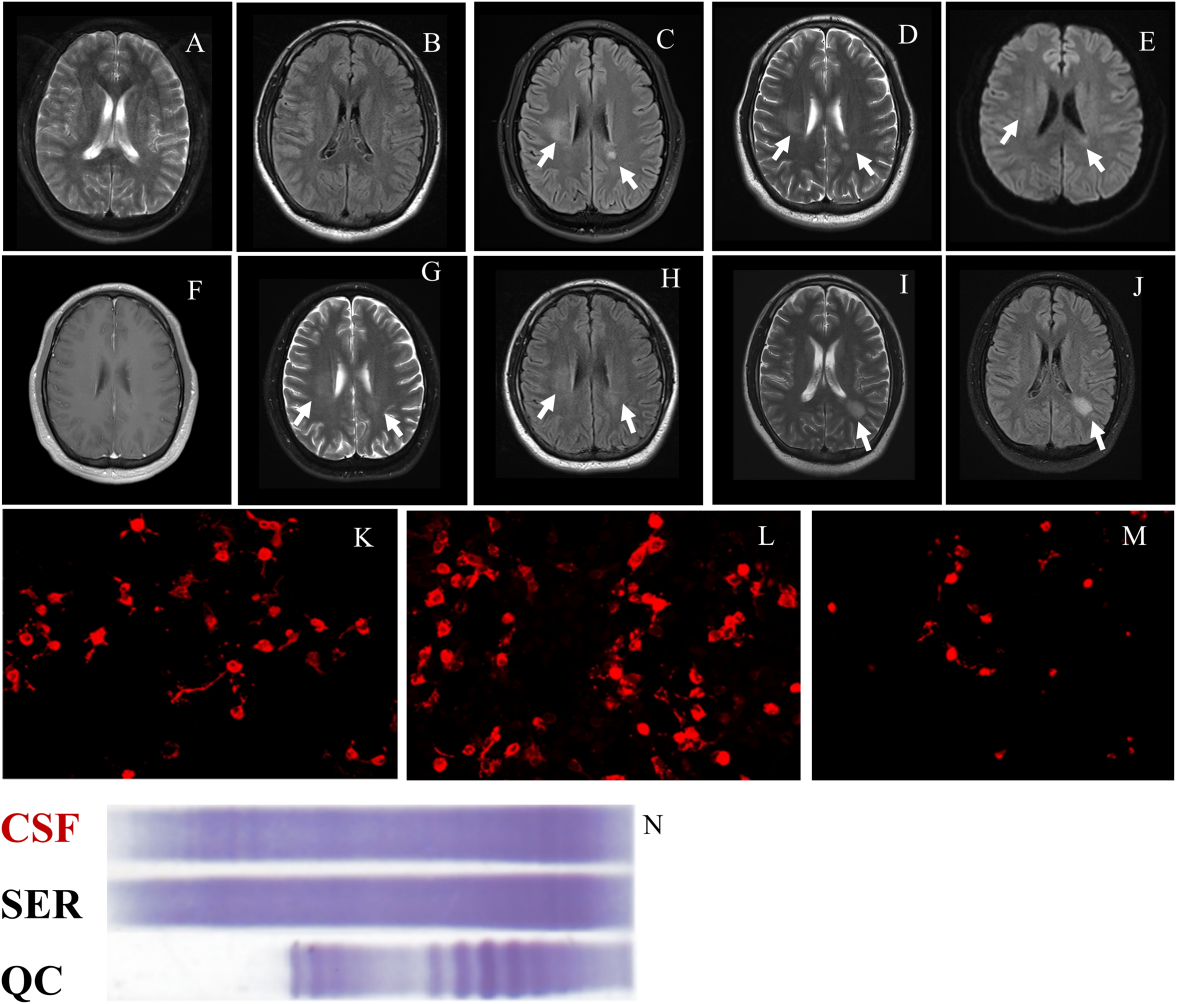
Brain MRI and antibodies in this case. (A) and (B) The MRI performed in October 2021 demonstrated normal results on T2 weight imaging and FLAIR imaging. (C–E) The MRI result showed significant hyperintensity in the right semioval center and the left posterior horn of the lateral ventricle on FLAIR imaging, T2 weight imaging, and DWI on June 1, 2022. (F) Enhanced magnetic resonance imaging performed on June 1, 2022, not showing a significant lesion. (G) and (H) The results of the MRI performed on June 1, 2022 demonstrated that the hyperintensity lesions were alleviated than before on T2 weight imaging and FLAIR imaging. (I) and (J) The results of the MRI performed on October 1, 2022 showed a new lesion with hyperintensity in the left posterior horn of the lateral ventricle on T2 weight imaging and FLAIR imaging. (K, L) Anti-NMDAR antibodies were found to be positive in serum (1:100) and in CSF (1:30) on June 1, 2022. (M) Anti-NMDAR antibodies were found to be positive in CSF (1:10). (N) OB was positive in CSF (counts, ≥2). CSF, cerebrospinal fluid; DWI, diffusion-weighted imaging; FLAIR, fluid-attenuated inversion recovery; MRI, magnetic resonance imaging; OB, oligoclonal immunoglobulin bands; anti-NMDAR, anti-N-methyl-D-aspartate receptor; QC, quality control; SER, serum.
